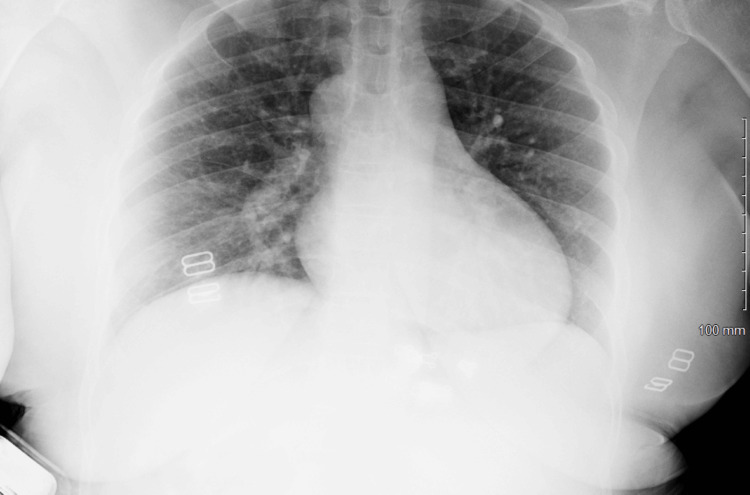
Chest X-ray obtained in the emergency department showing no acute process.
